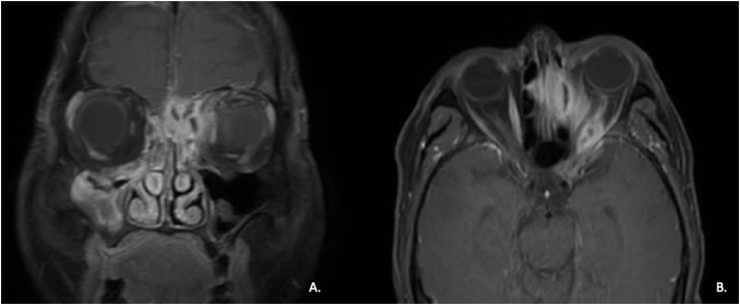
Gadolinium-enhanced T1-weighted magnetic resonance (MR) image. A, coronal view showing enhanced opacity of left frontal and ethmoidal sinuses and right maxillary sinus. B, axial view showing left ethmoidal sinus by solid mass and mucosal thickening with T1 hyperattenuation and extension to lacrimal duct and orbit, associated the presence of an intraconal abscess.
